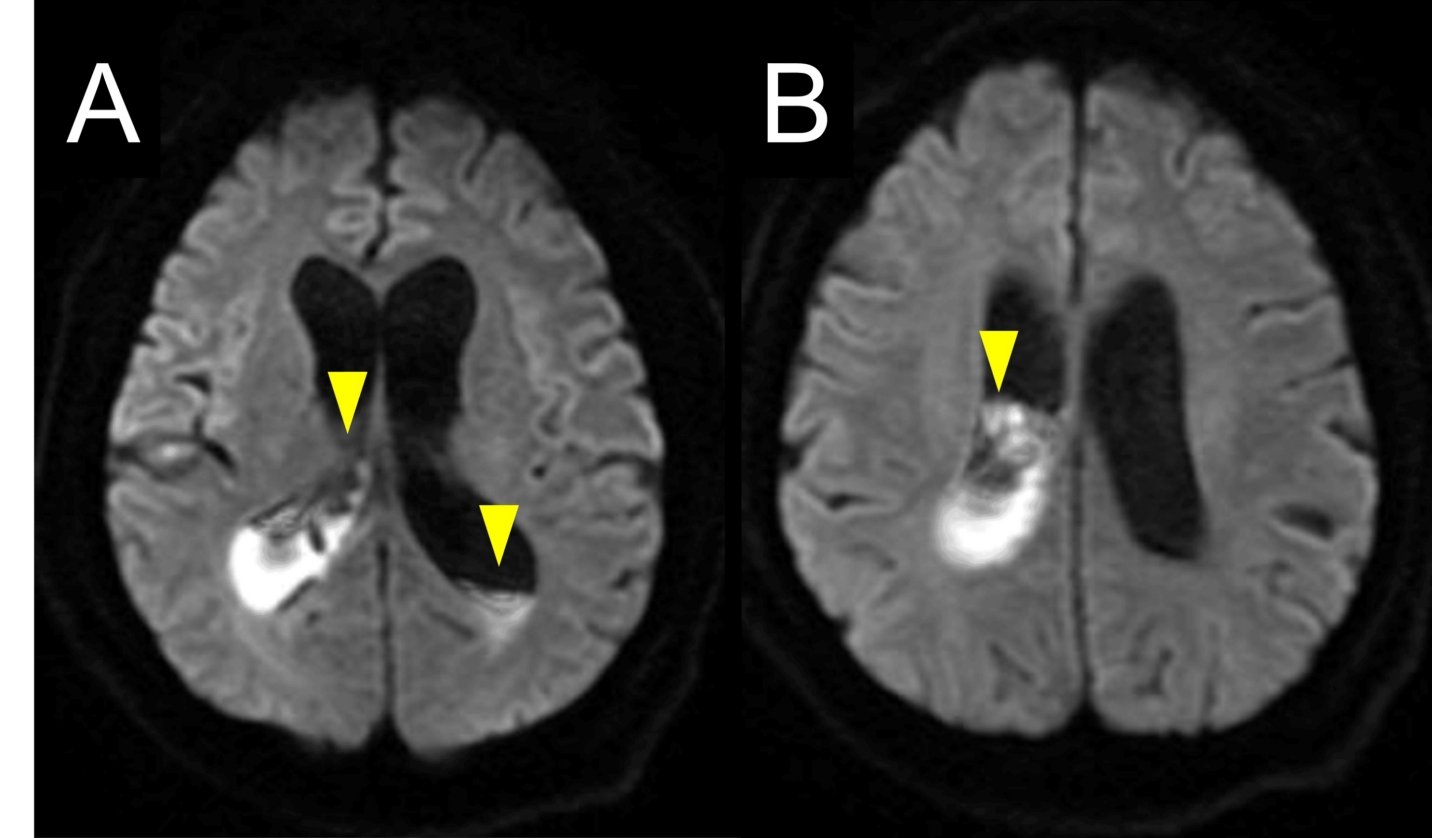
Brain MRI on day three. Diffusion-weighted imaging on day three showed high signal intensity in the lateral ventricles, consistent with intraventricular pus (yellow arrowheads).
